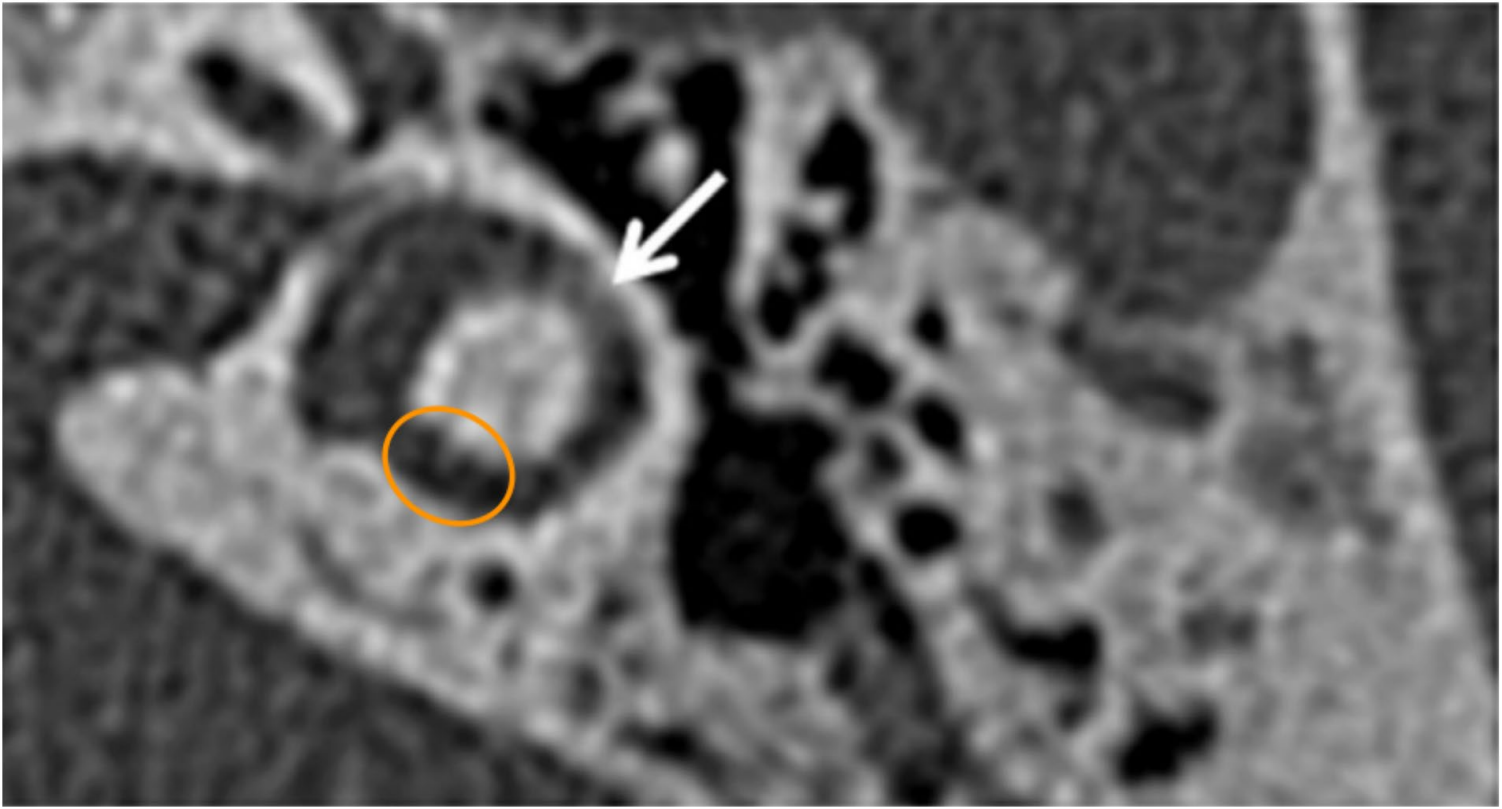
High resolution temporal bone CT of a normal left-sided horizontal semicircular canal. By convention in radiologic images, the top of the image is anterior, the bottom of the image is posterior, the left side of the image is the patient’s right, and the right side of the image is the patient’s left. The white arrow in the original image is merely indicating the horizontal semicircular canal. The orange ellipse highlights the posteromedial segment of the canal. From Oonk et al. [14]. Reproduced with written permission from Elsevier B.V.
